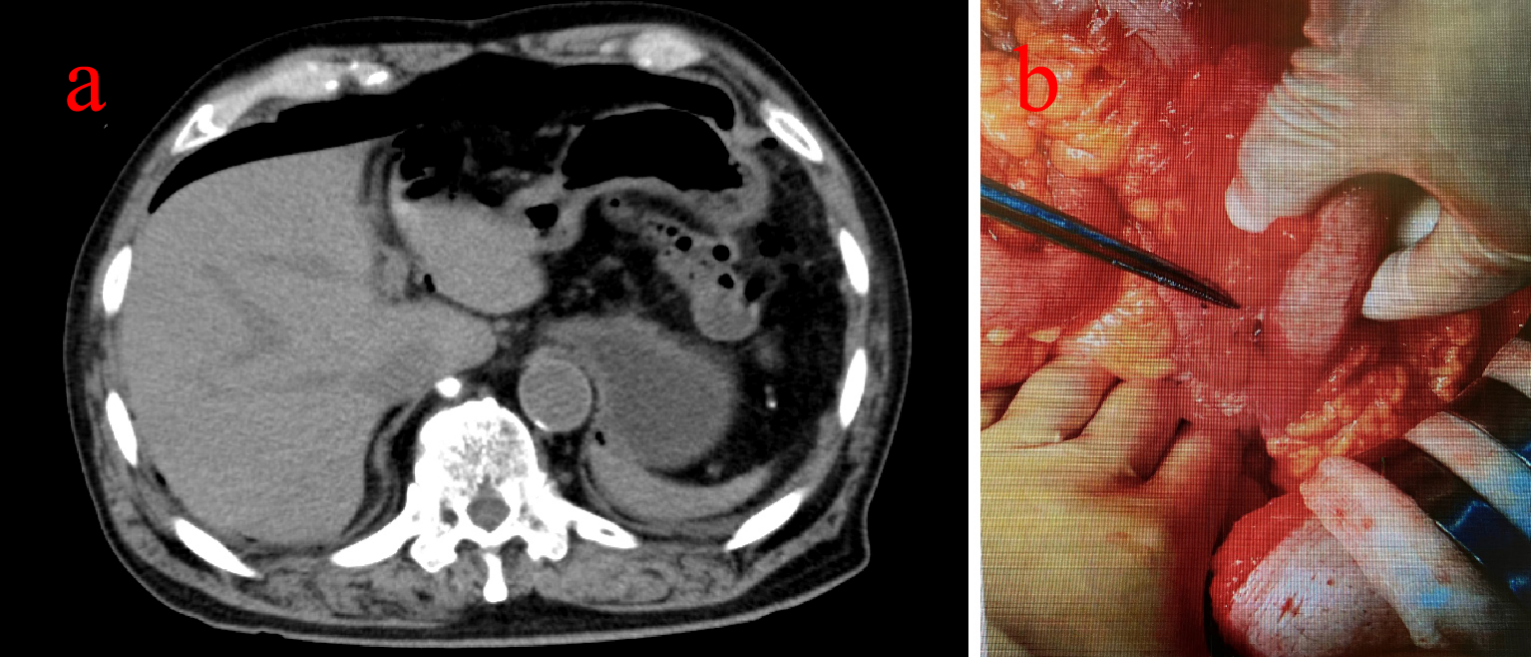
a. Computed tomography showed free air on the liver surface. b. We identified a 5 × 7 mm perforation in the transverse colon.
